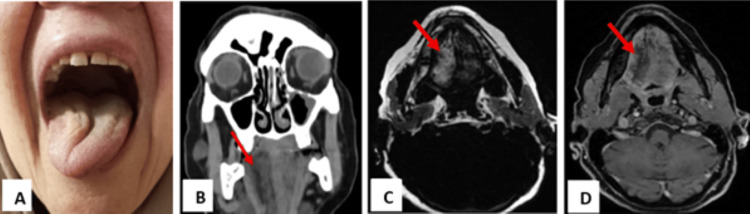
(A) Physical examination showing hemiatrophy of the tongue on the right side, and the inability to completely deviate the tongue toward the left side on protrusion. (B) Coronal reconstruction CT scan. (C) Axial T1 Dixon fat-only sequence in MRI. (D) Axial T1 fat-saturated imaging with contrast administration, showing fatty denervation of the right hemitongue (red arrow). CT scan: computed tomography scan; MRI: magnetic resonance imaging
